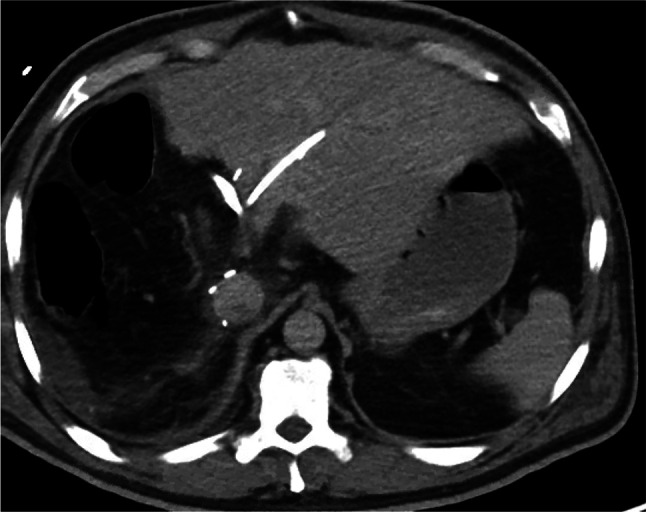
Computed tomography using a liver protocol following right hepatectomy showing appropriate postsurgical changes and biliary stent in left hepatic duct.
